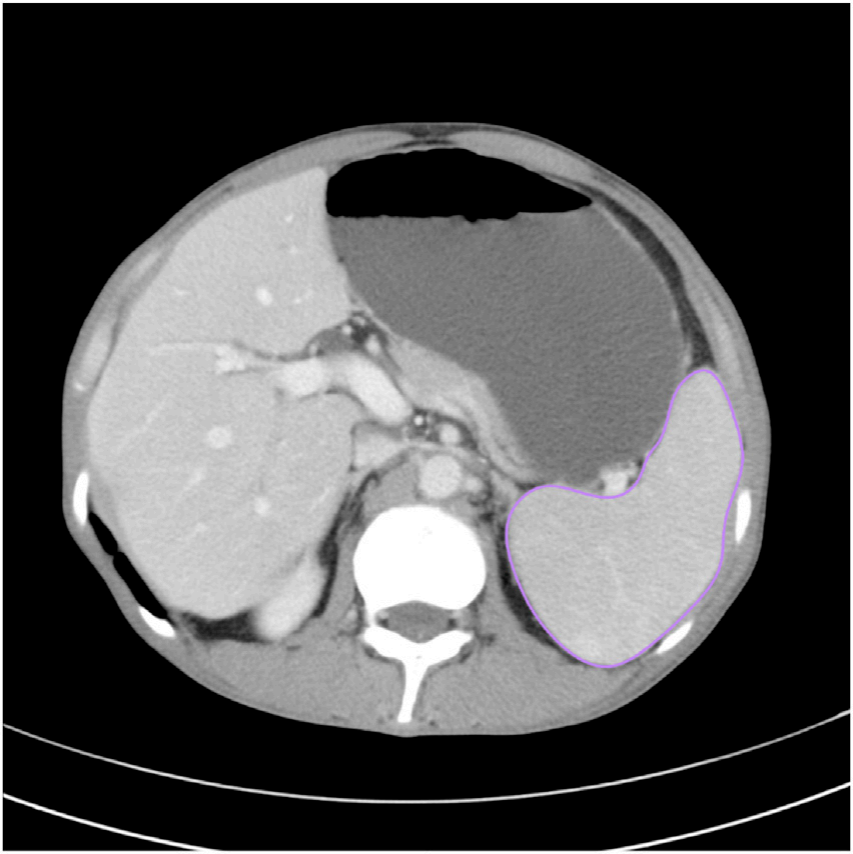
Spleen region of interest (ROI) on the CT slices to measure the splenic volume.
